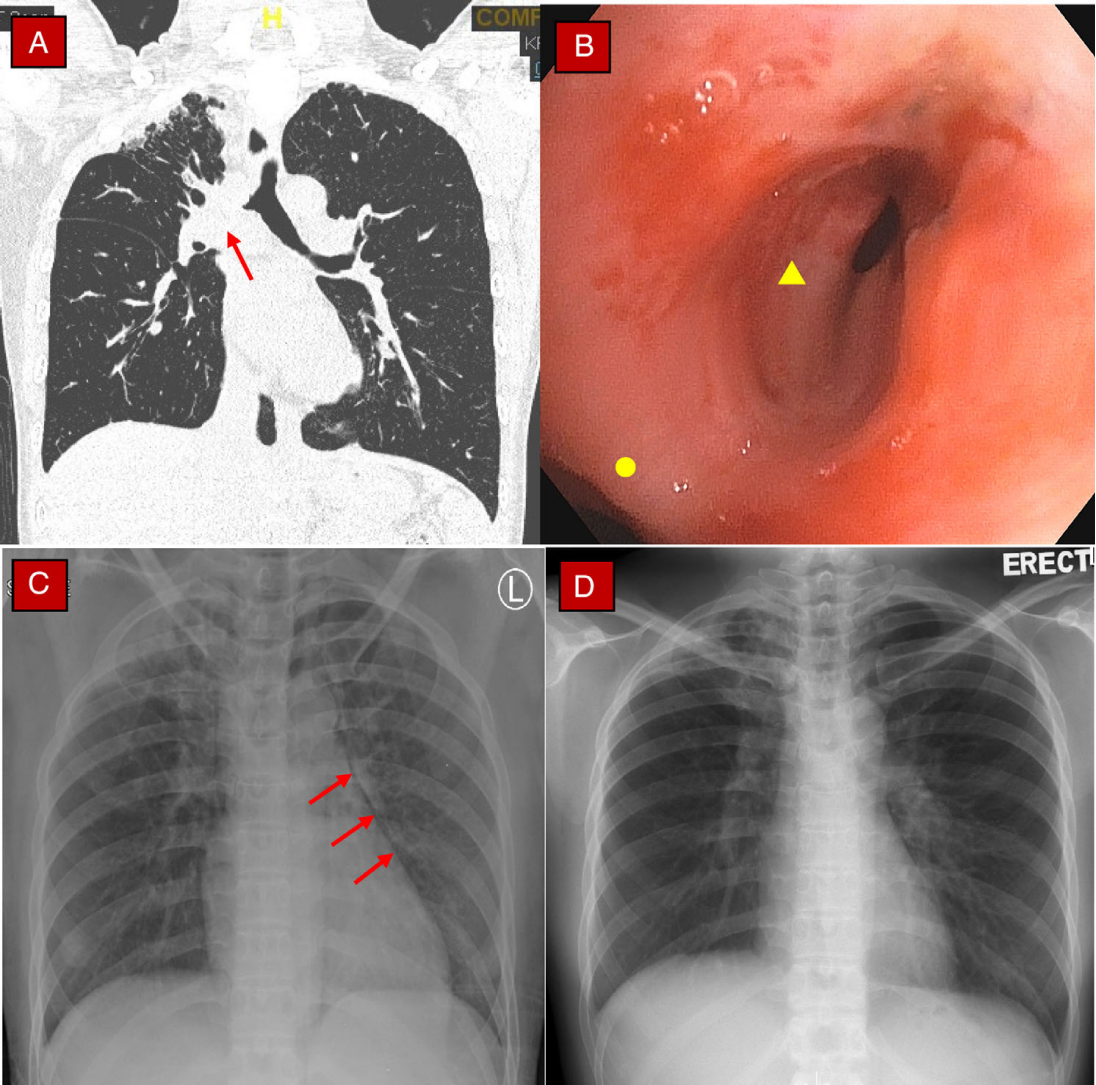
Computed tomography (CT), bronchoscopy image, and serial chest radiograph. (A) CT thorax demonstrating narrowing of the right main bronchus due to bronchomalacia (red arrow). (B) Bronchoscopy showing bronchomalacia of the right main bronchus (yellow triangle); main carina marked with yellow circle. (C) Chest radiograph done immediately post procedure revealing pneumomediastinum (red arrows). (D) Repeated chest radiograph 24 h later showing resolution of pneumomediastinum.
